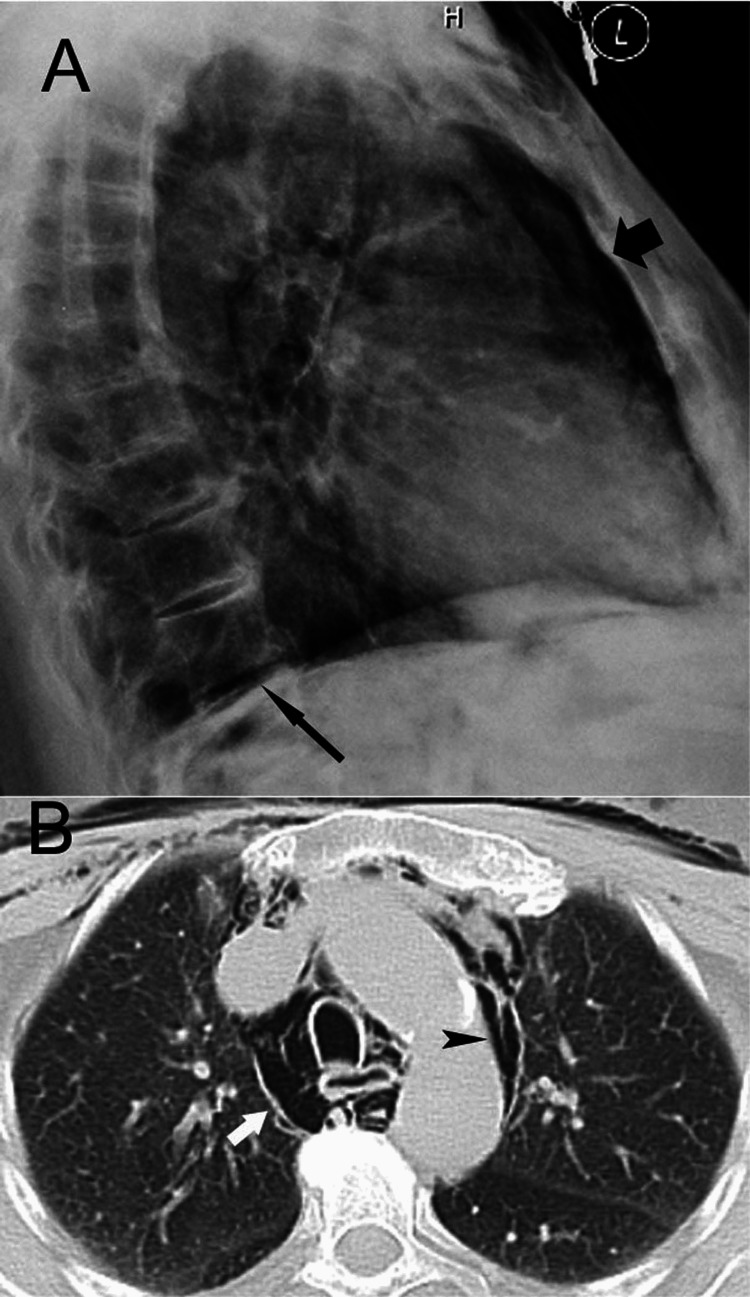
Scans of a 72-year-old female who underwent a colonoscopy that caused perforation of a diverticulum in the sigmoid A: A lateral chest radiograph shows pneumomediastinum (thick arrow) and pneumoperitoneum (long arrow); B: A CT image delineates pneumomediastinum (arrowhead) and pneumothorax (arrow).
